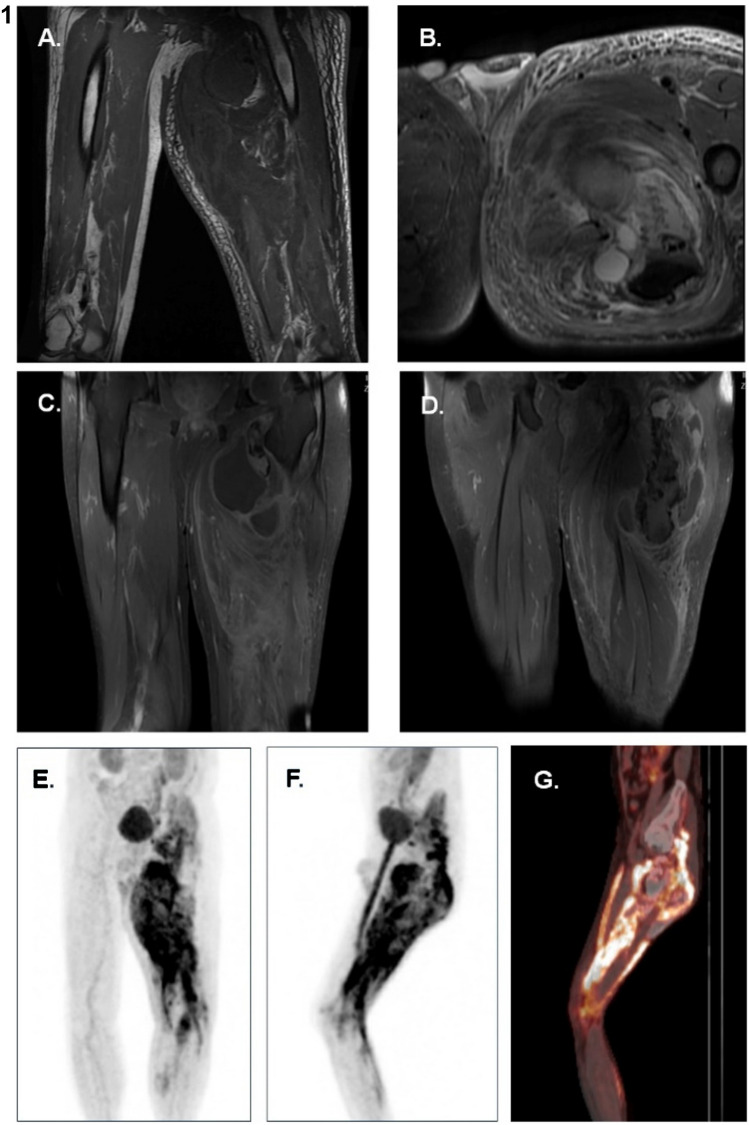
MRI Femur and FDG PET/CT Scan. MRI Femur showed a large heterogeneous mass with extensive edema and osseus matrix deposition in the left thigh, matching with areas of FDG avidity on FDG PET/CT Scan. Malignant degeneration could not be excluded, however, there was no evidence of distant metastases. A., B.: MRI Femur Without Contrast from admission, coronal and sagittal T1-weighted images. C., D.: MRI Femur With Contrast, T1-weighted images 1.5 months after initiation of methylprednisolone (3.5 months after initial presentation). E., F., G.: FDG PET/CT scan from admission – E. and F. are Maximal Intensity Projection (MIP) images, and G. is a fused sagittal image
